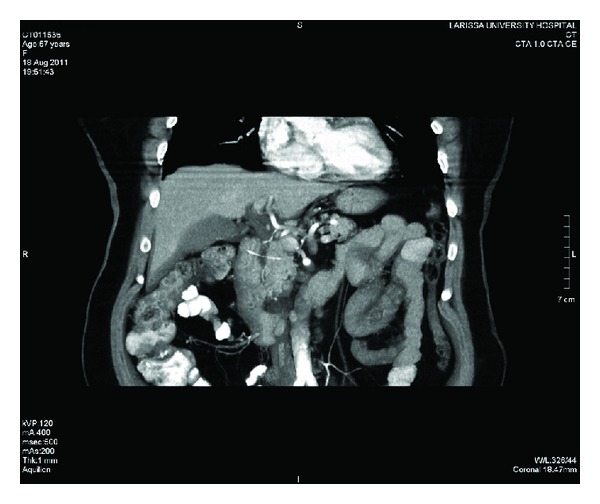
Contrast-enhanced CT image showing the radiopaque foreign body penetrating into the pancreatic head.
